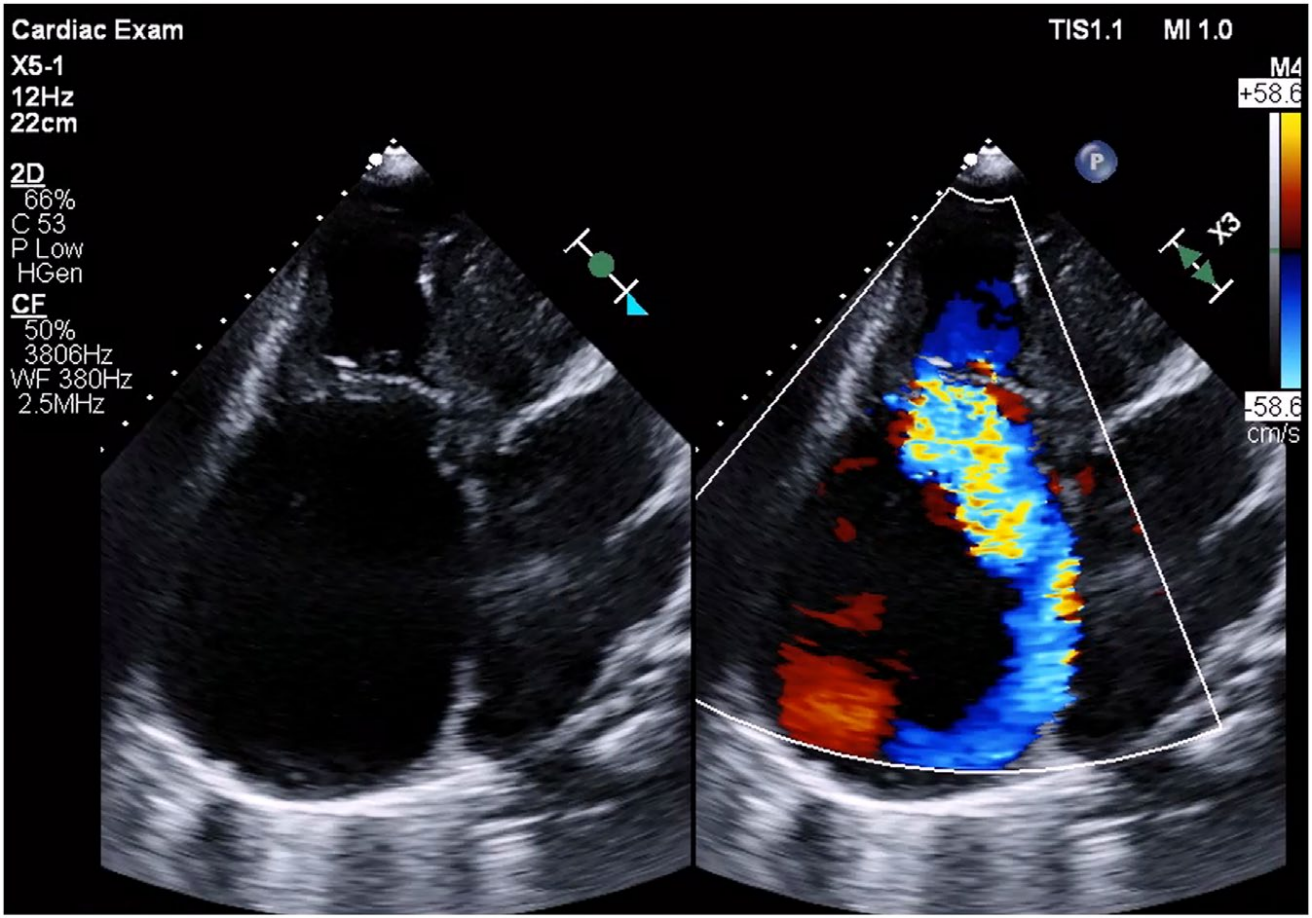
Echocardiography showing severe right atrial dilatation and severe tricuspid regurgitation.
